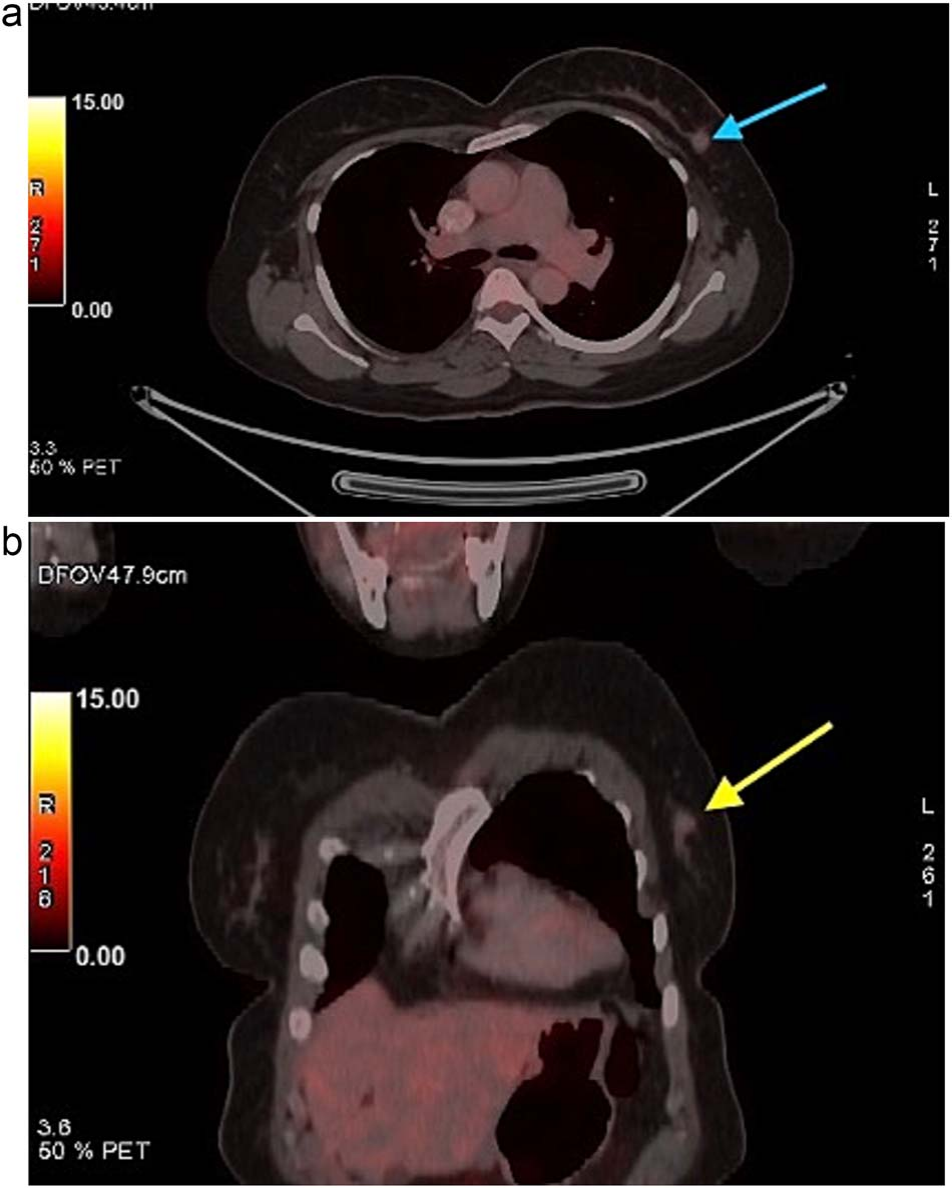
FDG PET CT images of the chest. (a) Axial PET CT image showing a well-defined FDG avid lesion in the upper outer quadrant of the left breast , consistent with the known carcinoma, with no evidence of axillary or mediastinal lymphadenopathy. (b) Coronal PET CT image demonstrating the left breast lesion in the upper outer quadrant without invasion into the chest wall or adjacent structures, consistent with localized disease.
